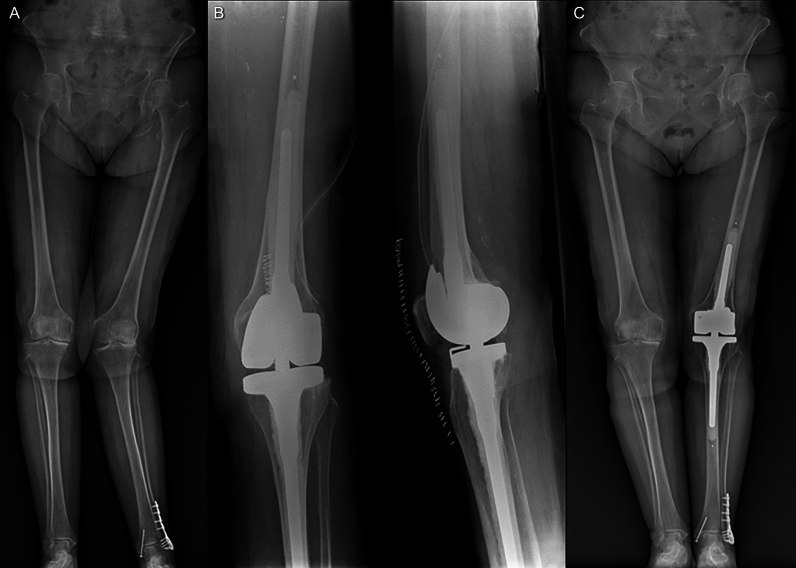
Case T.C., 84-year-old female; A The preoperative full weight-bearing X-ray shows left knee arthritis, more severe on the lateral side, with a severe valgus deformity. B Two projection knee X-ray shows the postoperative RX evaluation of the hinged implant. C
